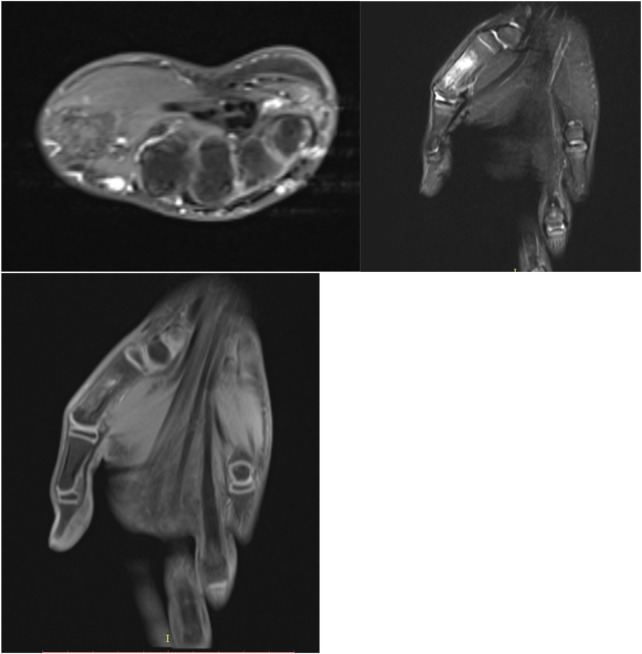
Preoperative MRI demonstrating diaphyseal tumor with thenar extension.
